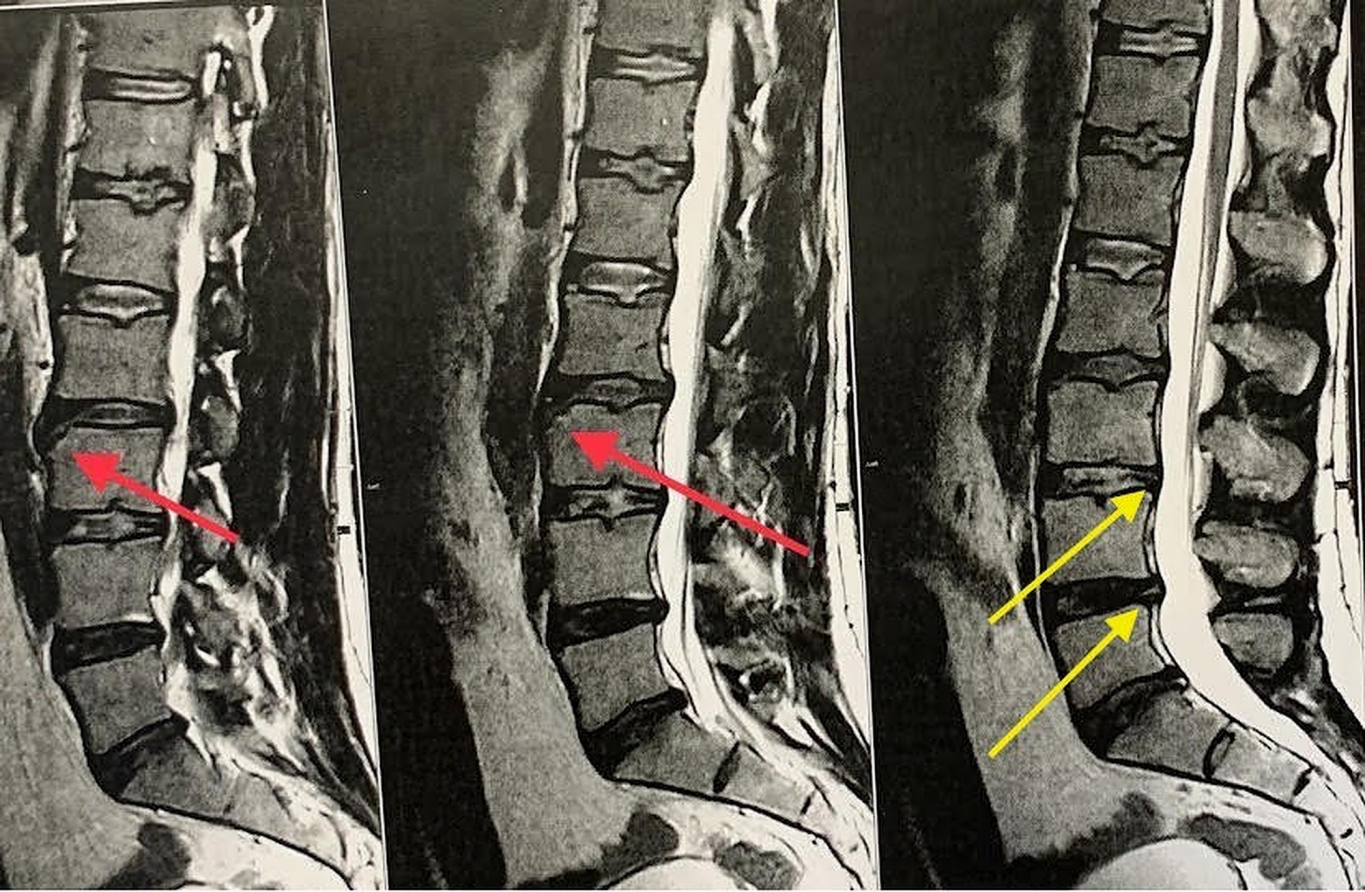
MRI scan, T2 sequence showing abnormal signal at the anterosuperior edge of L3 vertebra (red arrow) and adjacent intervertebral disc herniation at level L3-L4 and L4-L5 (yellow arrows).
